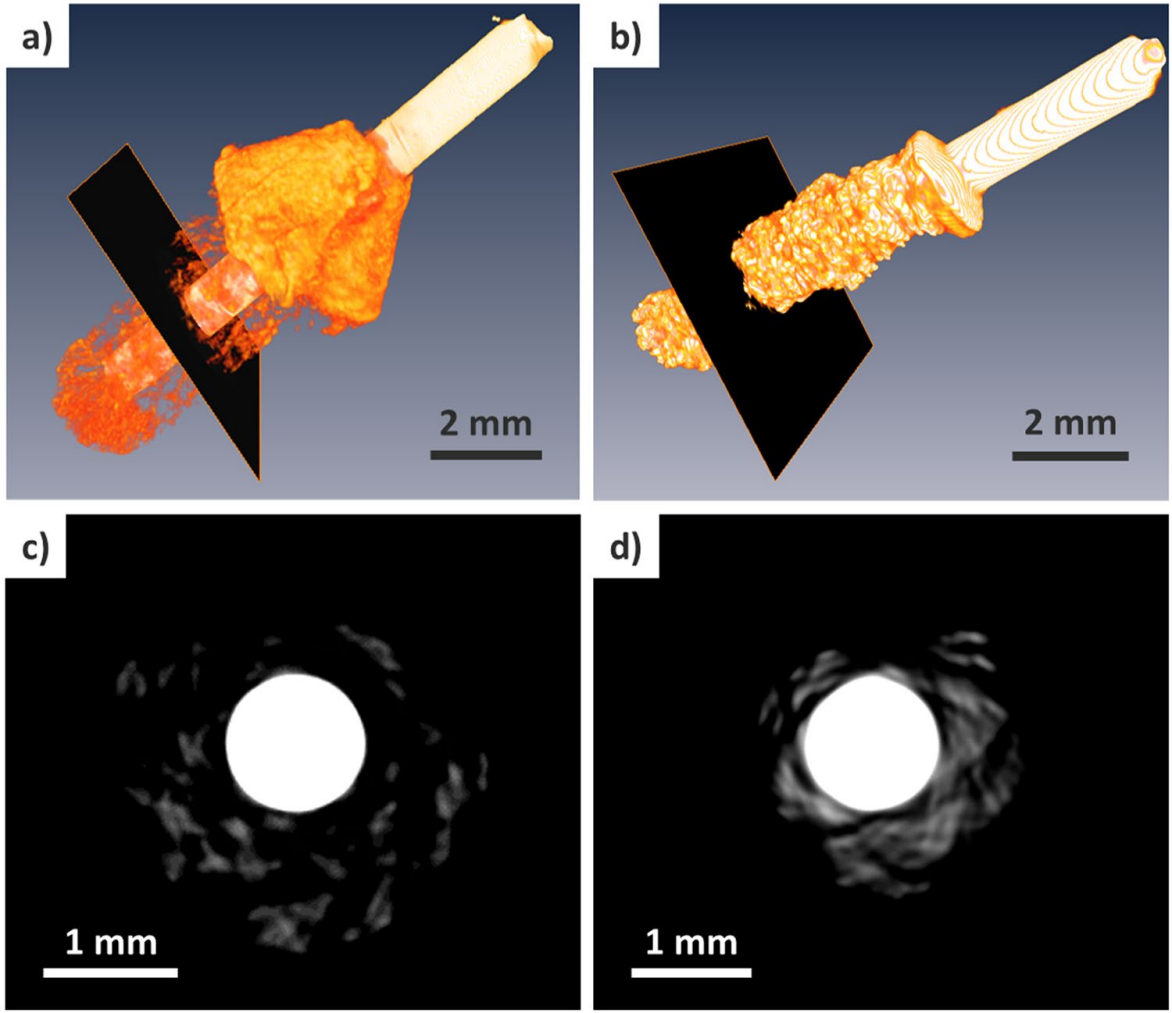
Reconstructed CT images, (a,b). Slice images of reconstructed electrodeposits, (c,d), described in Fig. 4.
